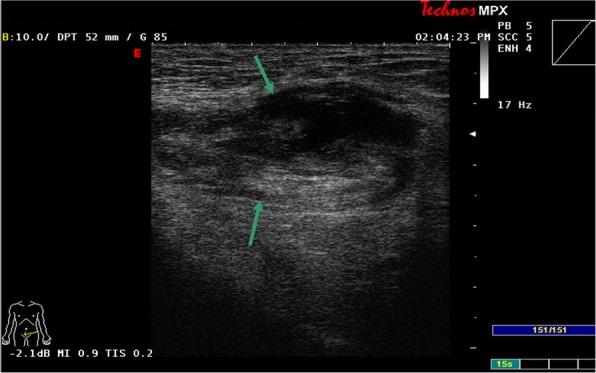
The hematoma showed in the ultrasonography. Arrows referred to the old hematoma
